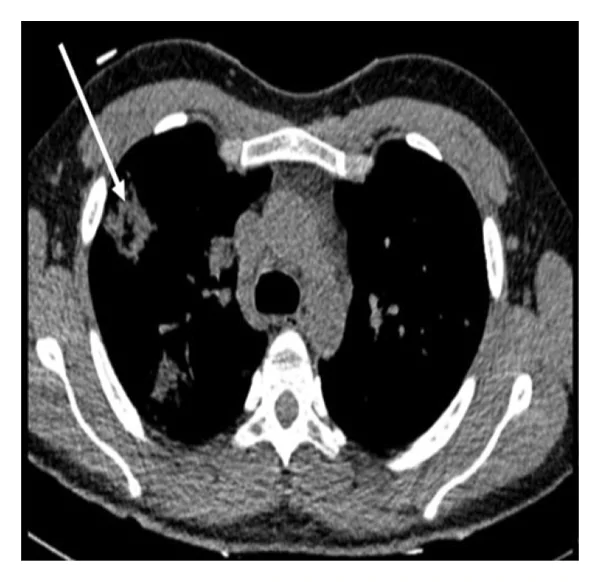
Contrast‐enhanced chest CT image showing multiple bilateral cavitary pulmonary lesions consistent with septic emboli.
